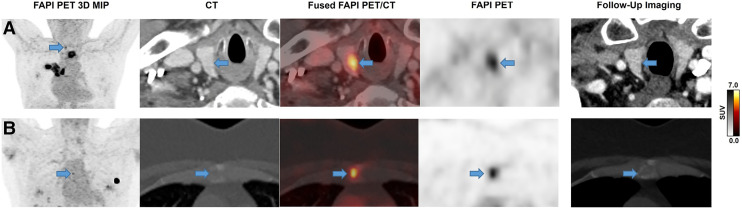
Row A depicts FAP-RADS 3A lesion in 73-y-old patient with non–small cell lung cancer with moderate focal FAPI uptake in right lobe of thyroid. There was no morphologic correlate on conventional follow-up imaging 6 mo later, leading to retrospective classification as unspecific FAPI uptake. Row B shows focal moderate FAPI uptake in manubriosternal joint with accompanying nonspecific CT correlate, meeting FAP-RADS 3B criteria, without any change on follow-up imaging 9 mo later. MIP = maximum-intensity projection.
